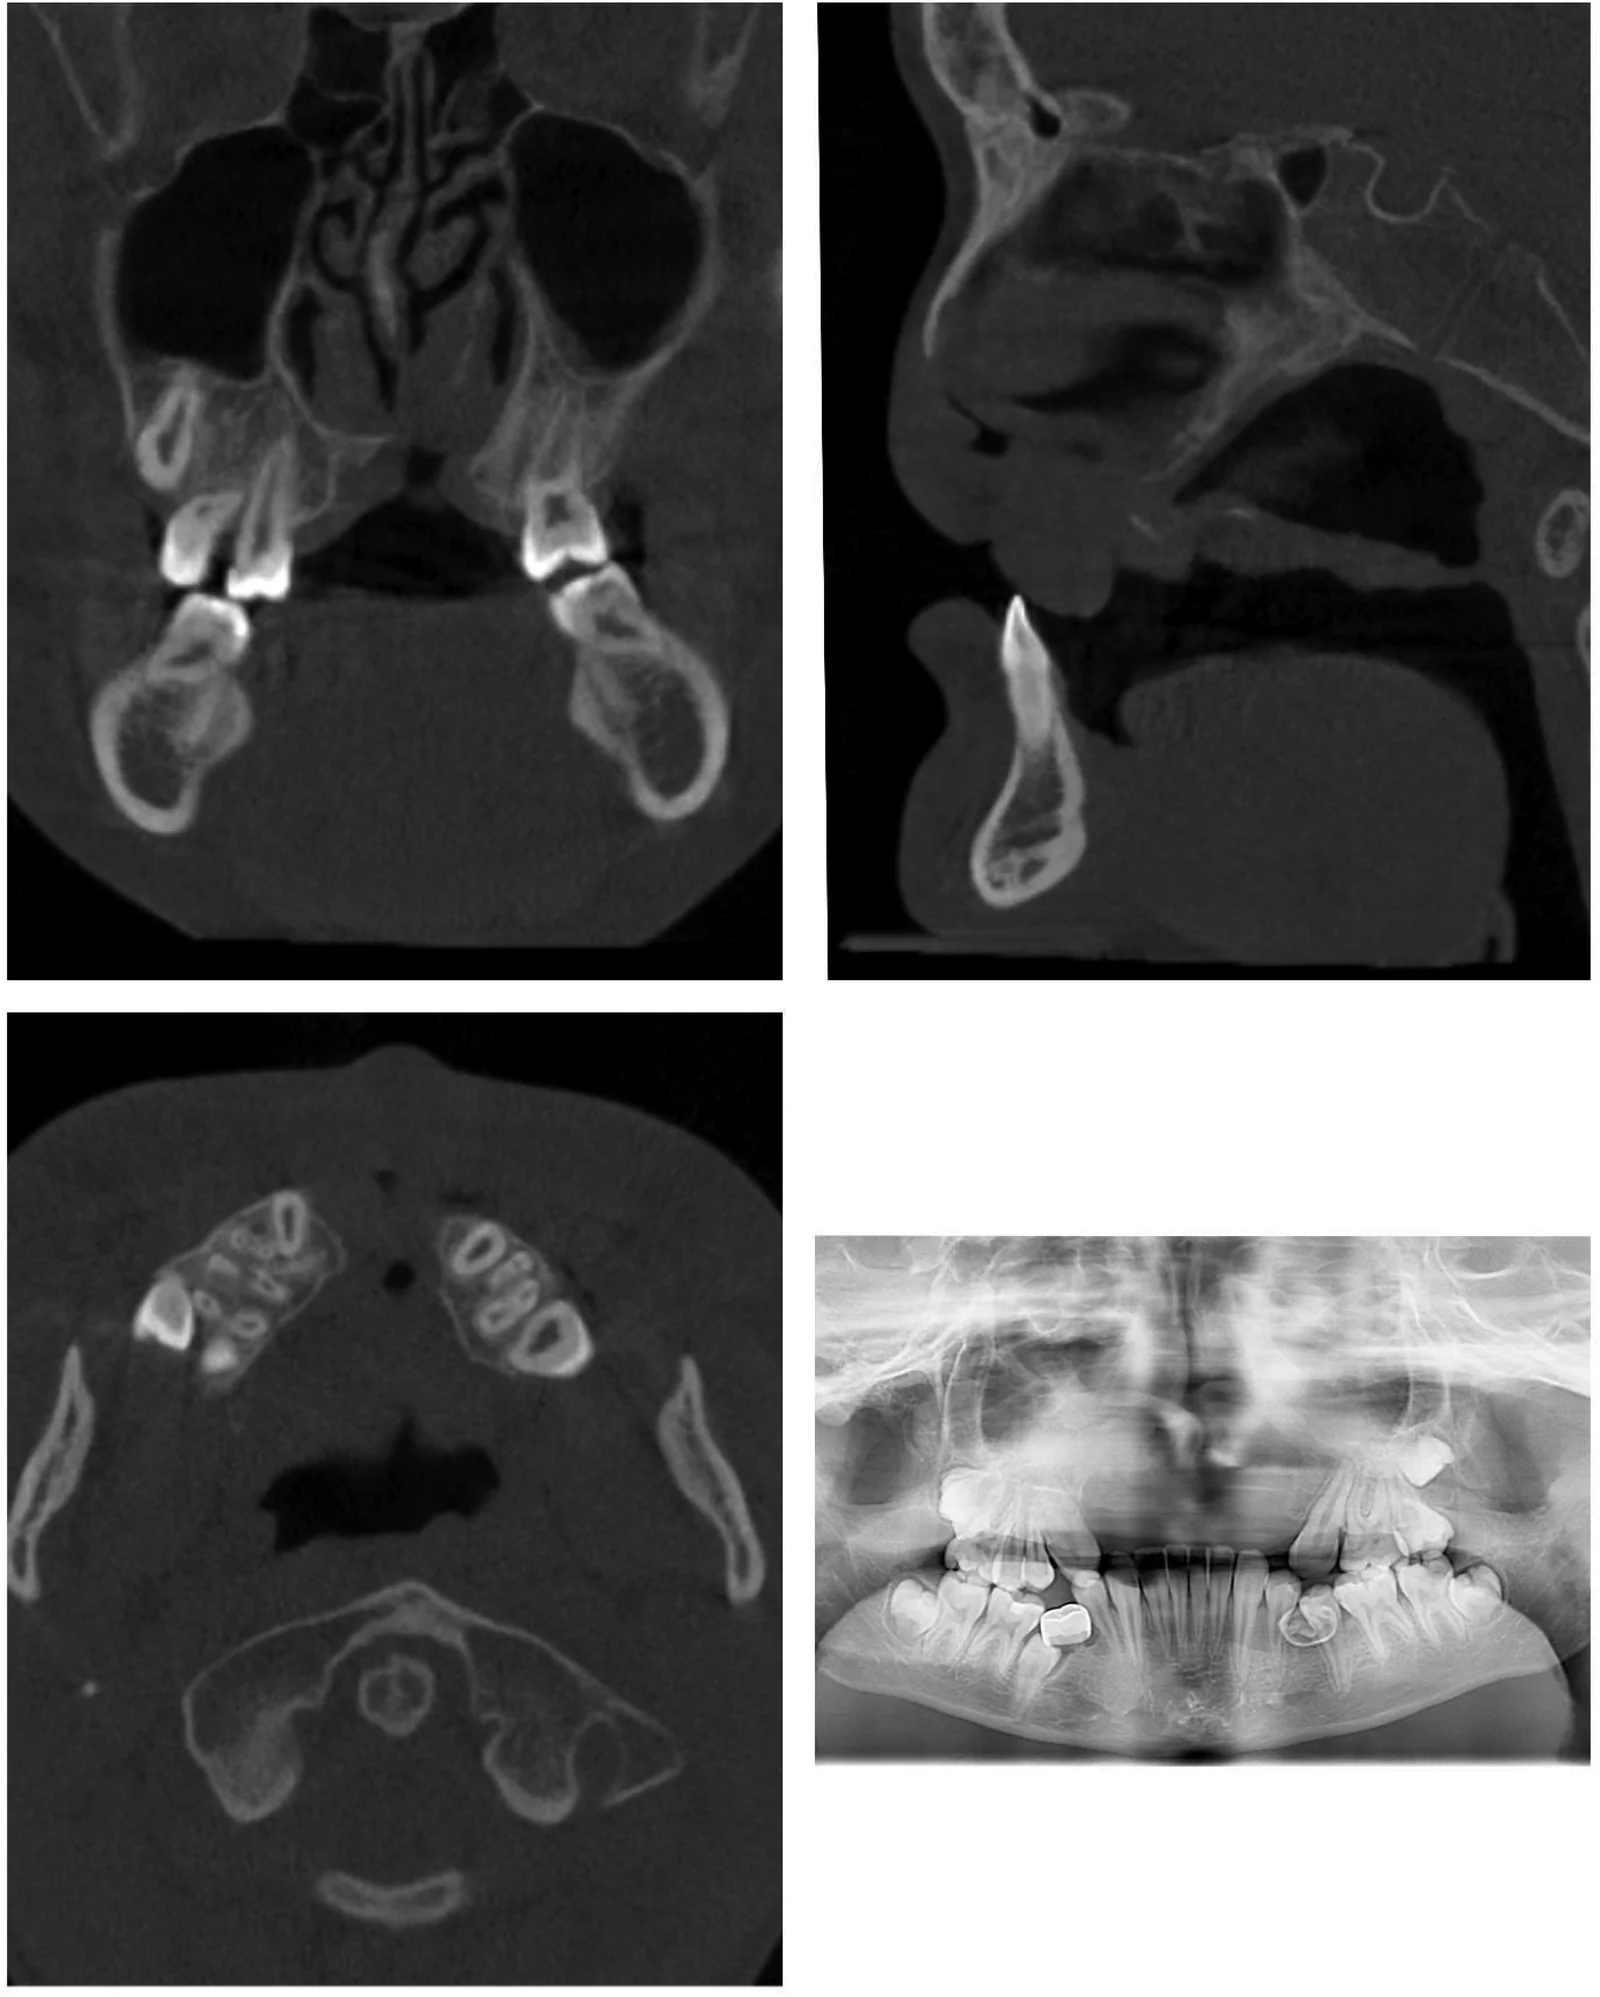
CT scan with single cuts from coronal (upper left), sagittal (upper right), axial (lower left), and panoramic views (lower right). Scans taken pre-procedure demonstrating missing premaxillary segment.
